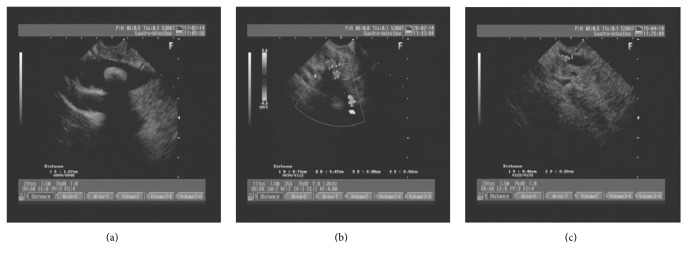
The diameter of common bile duct stones measured on linear endoscopic ultrasound. (a) >1 cm, (b) 0.5–1.0 cm, and (c) <0.5 cm.
